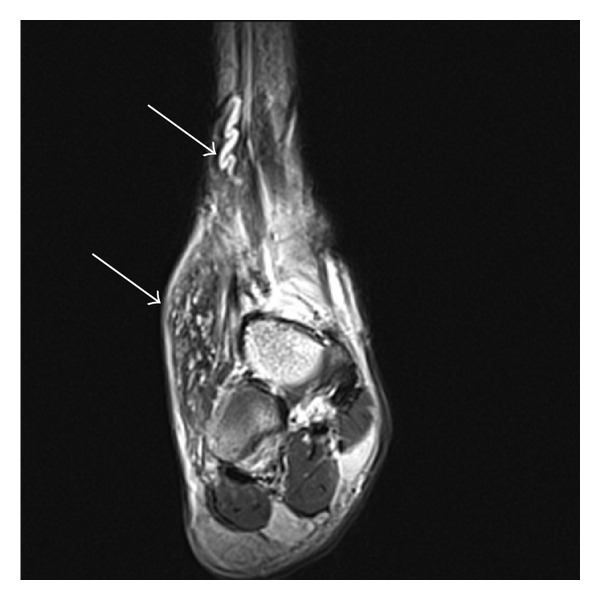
Coronal T1 MRI of right foot large subcutaneous soft tissue lesion anterolaterally (arrow) with a large prominent proximal feeding vessel (arrow).
